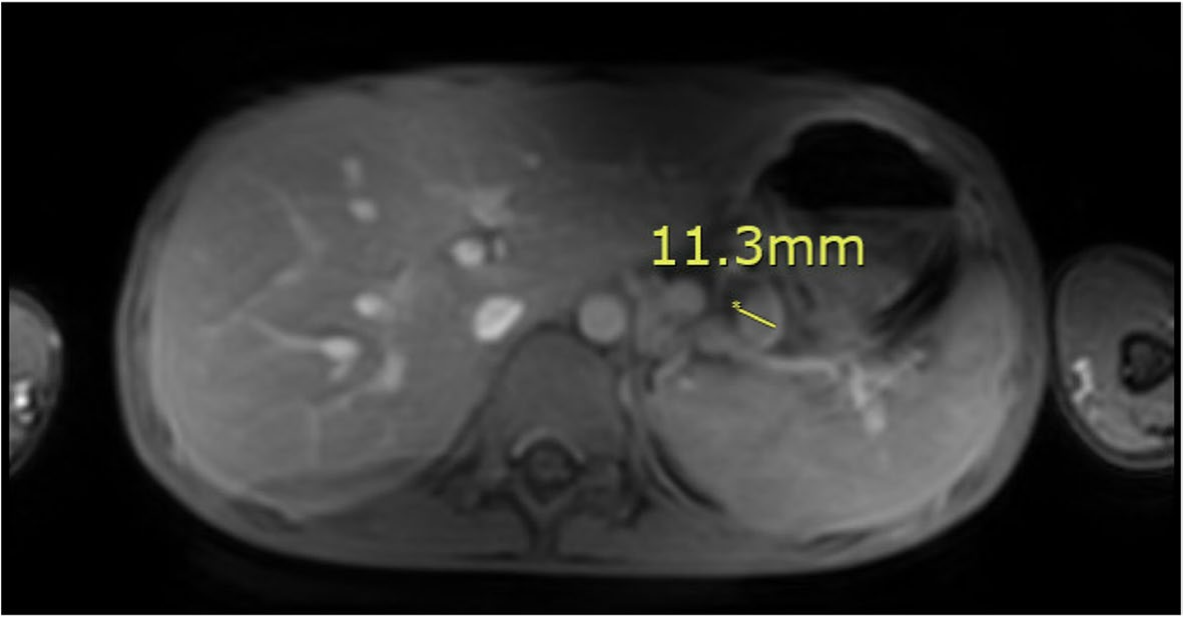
MRI with contrast showed an 11.3 mm lesser sac lymph node, one of the numerous enlarged lesser sac lymph nodes and circumferential nodes around the gastric cardia
